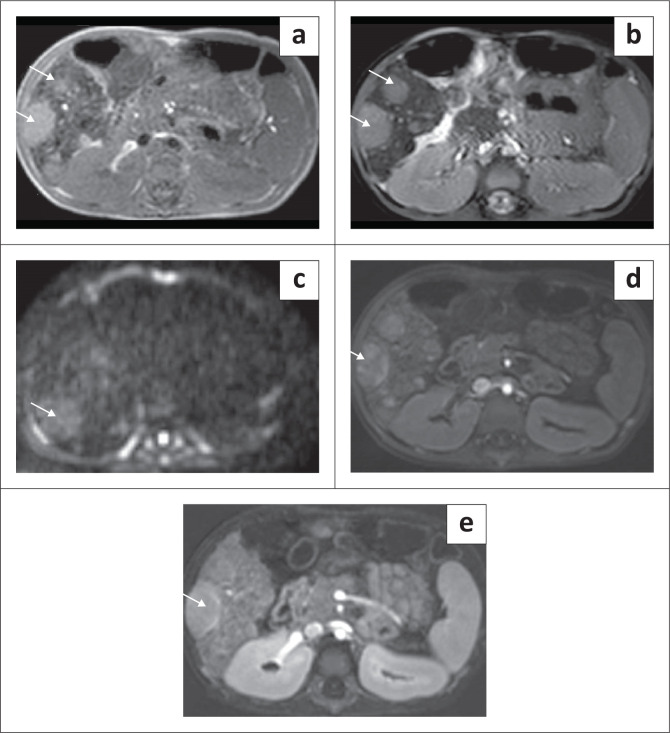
MRI of multiple liver adenomas in a child with glycogen storage disorder. Axial T1-weighted (T1W) (a) and T2-weighted (T2W) fat saturated (b) images of the upper abdomen of a 7-year-old child showing a few hyperintense lesions (arrows) in the right lobe of liver. The background liver intensity is abnormal, and the liver has nodular outline. Diffusion weighted trace image (c) showing mild restricted diffusion in the lesion (apparent diffusion coefficient [ADC] not shown). Dynamic contrast-enhanced images showing arterial enhancement (d) of the lesions (arrow) with retention of contrast in the portal venous phase (e).
